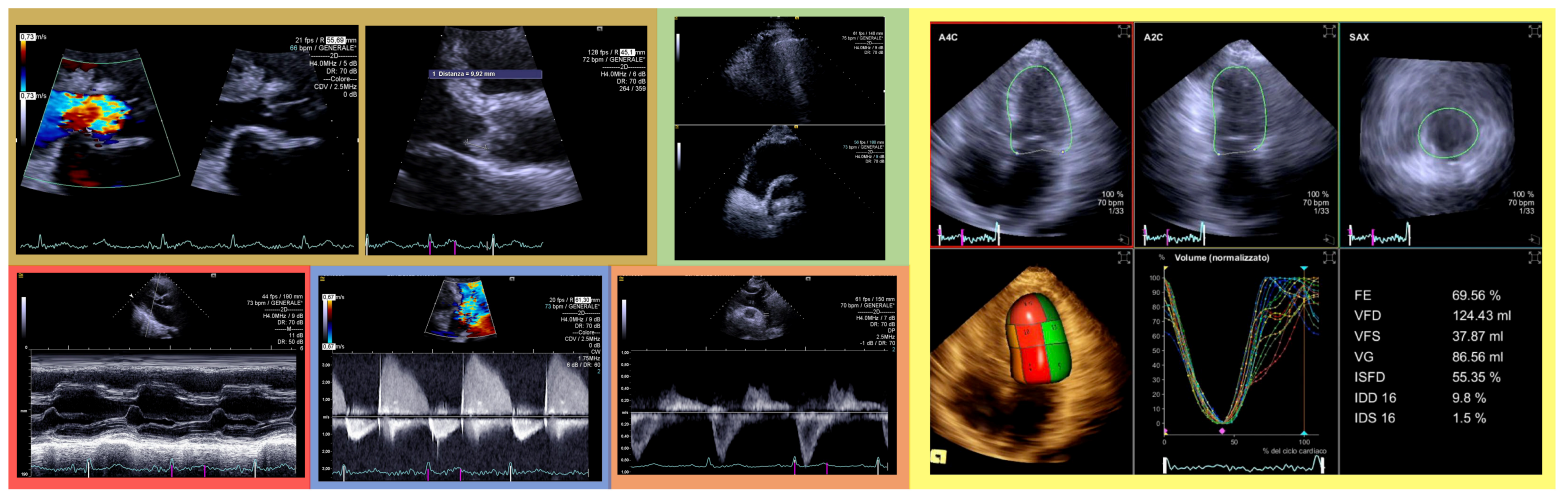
Brown squares: on the left, Color Doppler shows aortic
